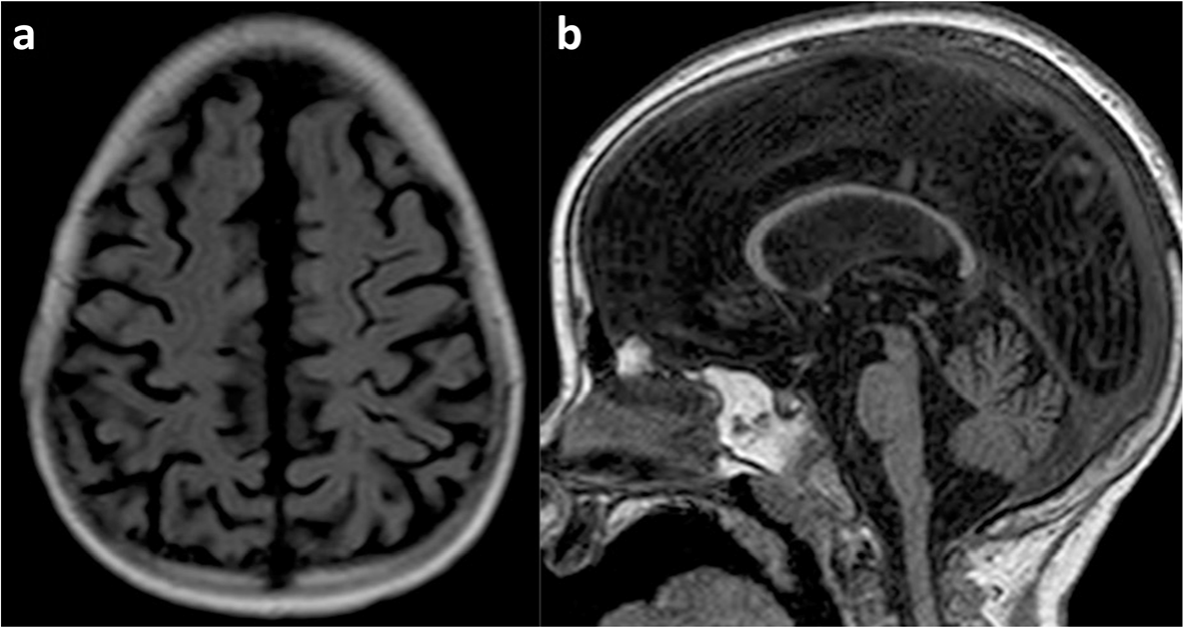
a, b Generalised atrophy, of unknown cause, in a 5-year-old girl. Axial FLAIR image (a) demonstrates generalised atrophy without any regional predominance in the watershed distribution and there is no ulegyria. The midline sagittal T1 image (b) demonstrates that there is diffuse moderate thinning of the corpus callosum but also without any regional predominance
